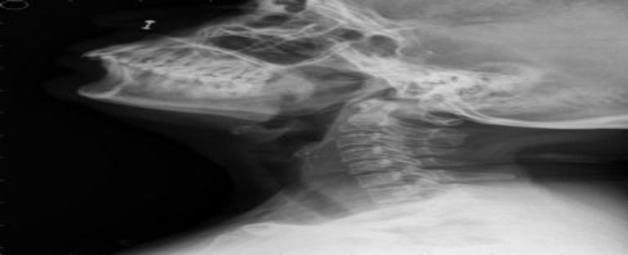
Lateral X-ray of the neck showing a radiopaque ingested foreign body, 3.5 cm, in the upper cervical esophagus with the sharp end in the neural foramen at the C5-6 level
